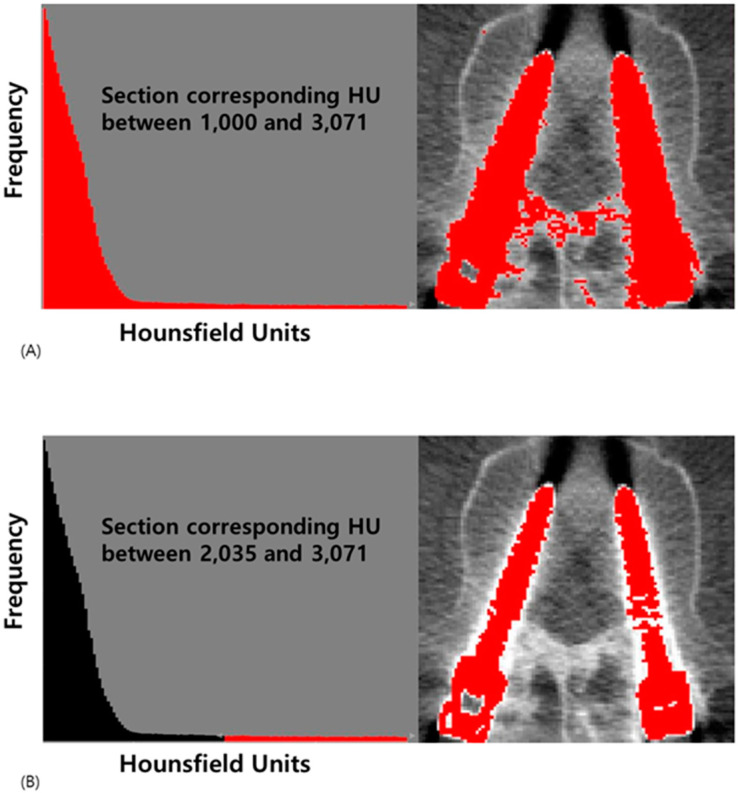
Hounsfield unit histograms of the axial CT images corresponding to the regions of interest. (A) The section corresponding to a HU value between 1000 and 3071 is highlighted (B) The section corresponding to a HU value between 2035 and 3071 is highlighted.
